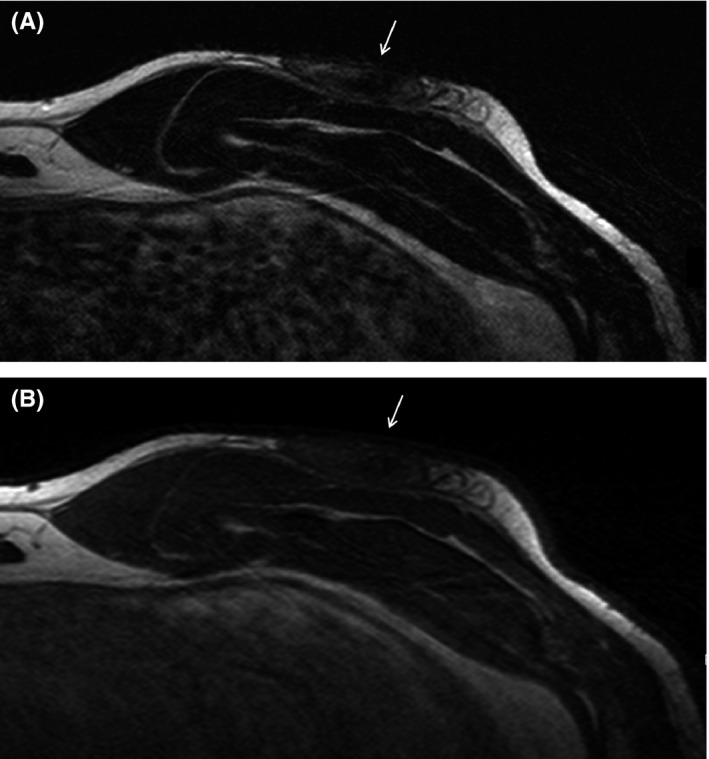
On T2‐weighted MR imaging (A) and T1‐weighted imaging (B), the lesion was identified as heterogeneous low signal intensity mass (arrows)
